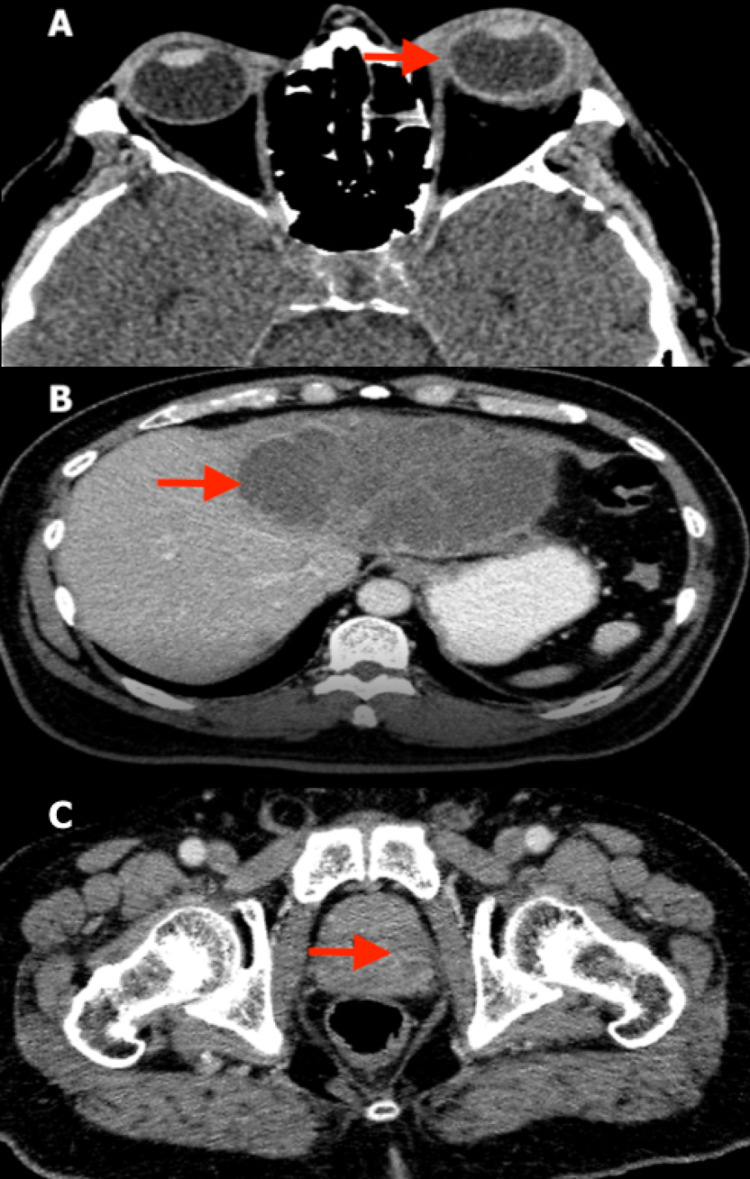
Contrast-enhanced computed tomography (CECT) scans (A) CECT orbit shows left proptosis with thickening and enhancement of the sclera. There is also enhancement of the periorbital soft tissue. Overall findings suggest left panophthalmitis. (B) CECT abdomen shows multiloculated rim-enhancing hypodense collection involving mostly the left lobe of the liver suggestive of liver abscess. (C) CECT pelvis shows hypo enhancement in the left lobe of the prostate suggestive of an abscess.
